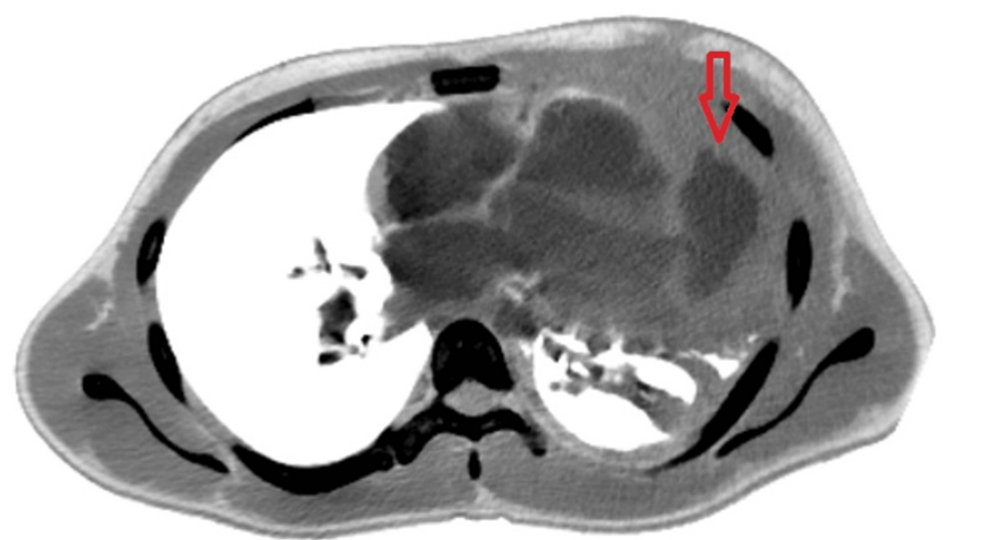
Chest CT scan showing the pseudoaneurysm (pointed by the red arrow) communicating with the left ventricular cavity through a narrow neck.
